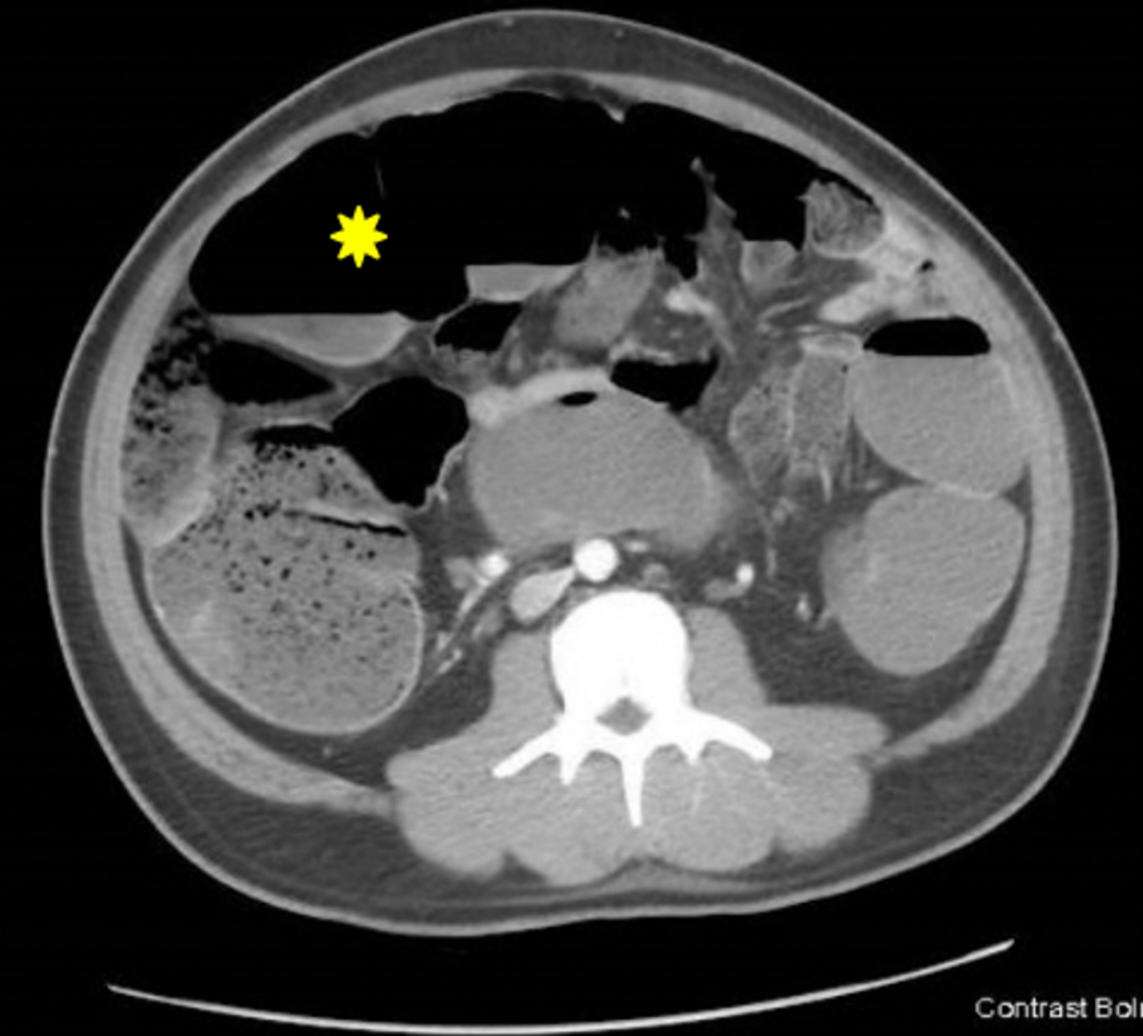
Transverse CT section demonstrating large bowel obstruction (yellow asterisk).
